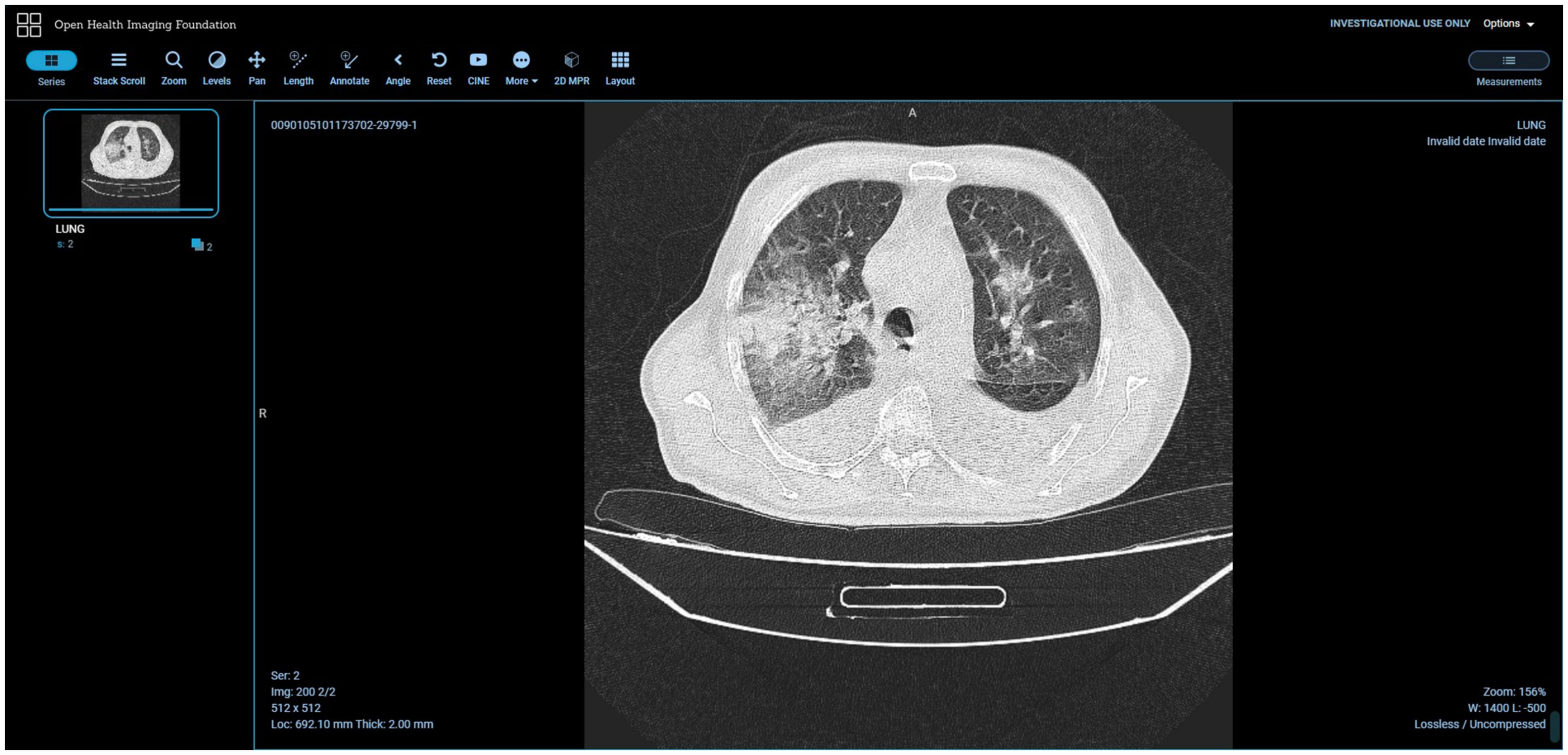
OHIF external viewer. Adapted with permission from “COVID19-CT-Dataset: An Open-Access Chest CT Image Repository of 1000+ patients with confirmed COVID-19 diagnosis” by Sayyed Mostafa Mostafavi, licensed under CC0 1.0. COVID19-CT-Dataset: An Open-Access Chest CT Image Repository of 1000+ patients with confirmed COVID-19 diagnosis. CT images of subjects with confirmed lung infections after positive Covid-19 diagnosis.
